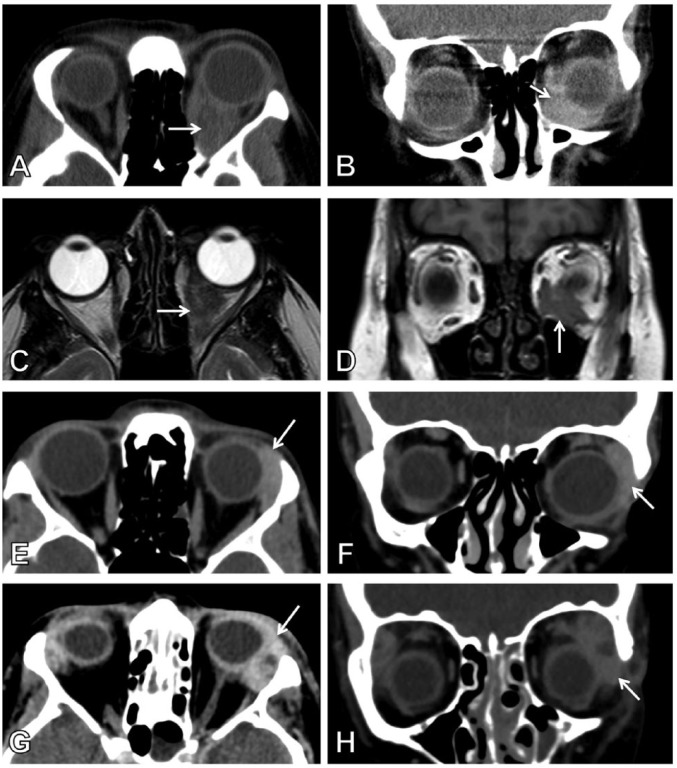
Orbital imaging in the 3 cases. Arrows indicate the site of the inflammatory masses. (A, B) Axial and coronal CT reformats of case 1. (C, D) Axial and coronal MRI of case 1. (E, F) Axial and coronal CT reformats of case 2. (G, H) Axial and coronal CT reformats of case 3. CT indicates computed tomography; MRI, magnetic resonance imaging.
